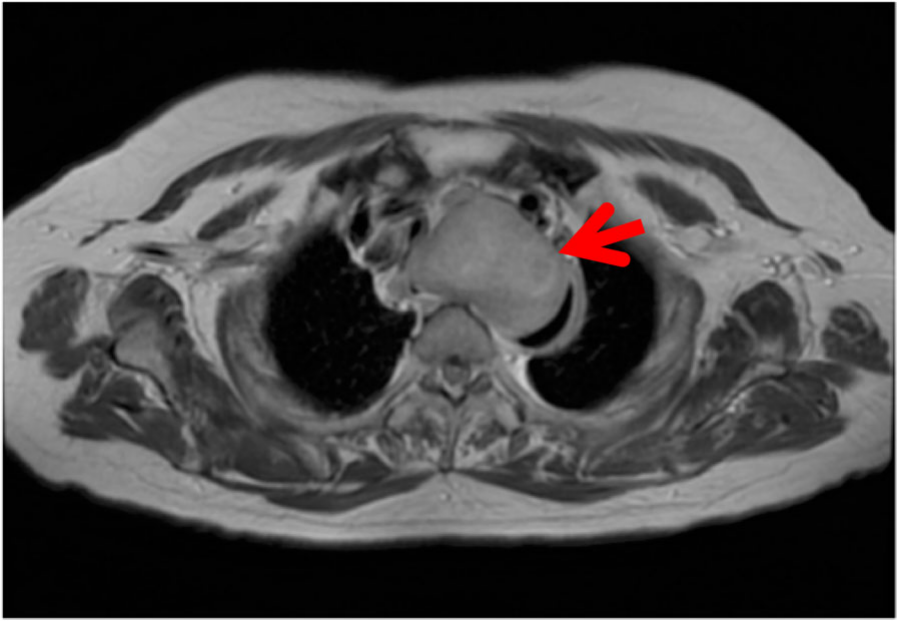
T2WI magnetic resonance imaging revealed a heterogeneous inner structure
